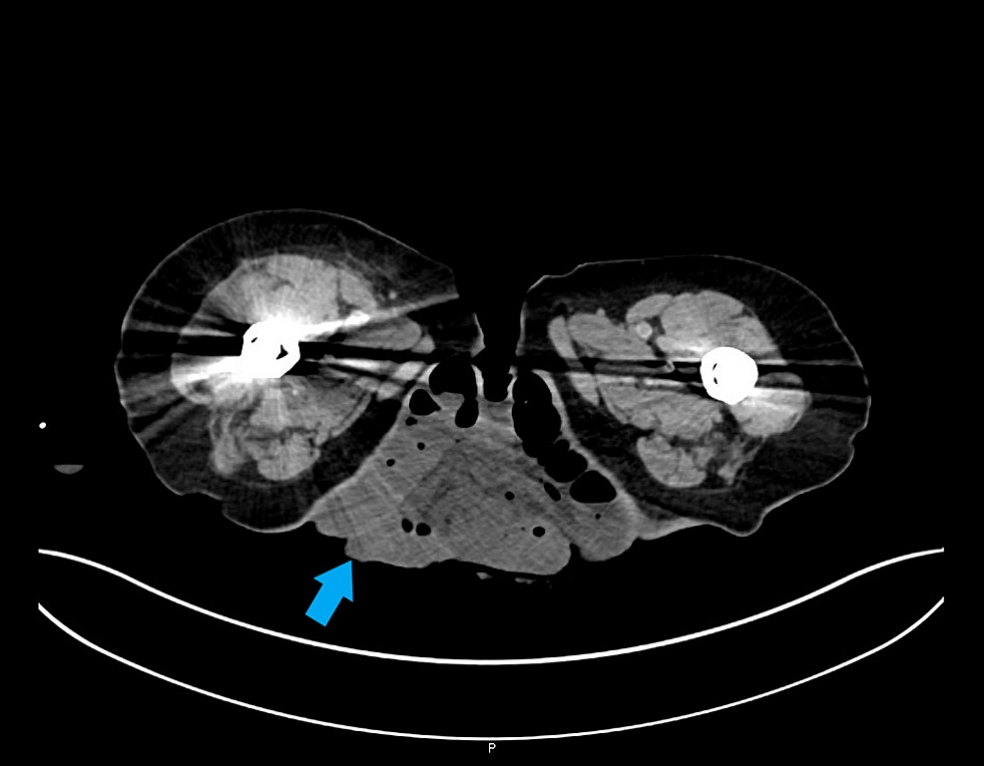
Axial CT abdomen and pelvis scan, showing the external herniated loops of small bowel.
